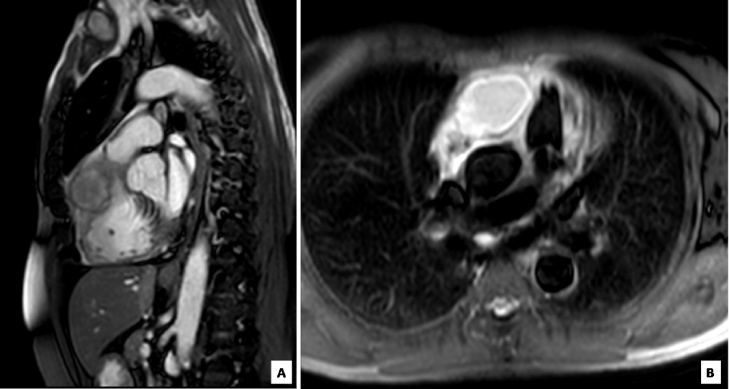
Sagittal (a) and transverse (b) magnetic resonance images of the heart showing a well-defined epicardial cystic lesion with a lobulated outline and thin internal septations.
